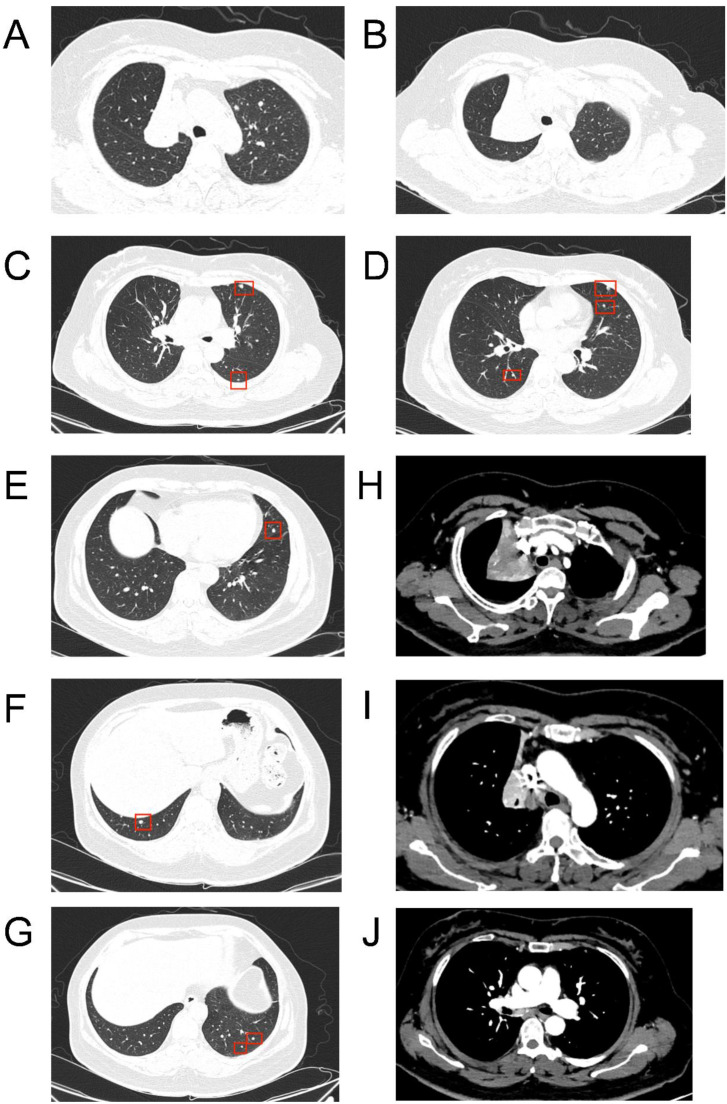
CT images at the patient’s initial visit. (A) Complete atelectasis of the right upper lobe; (B) Obstruction of the right upper lobe bronchus; (C) Multiple solid nodules in the left upper lobe; (D–G) Multiple solid nodules in both lungs; (H) Mass-like soft tissue density shadow in the right hilar region, showing marked heterogeneous enhancement with ill-defined borders on contrast-enhanced imaging; (I) Multiple small lymph nodes visible within the mediastinum; (J) Multiple small mediastinal lymph nodes are visible.
